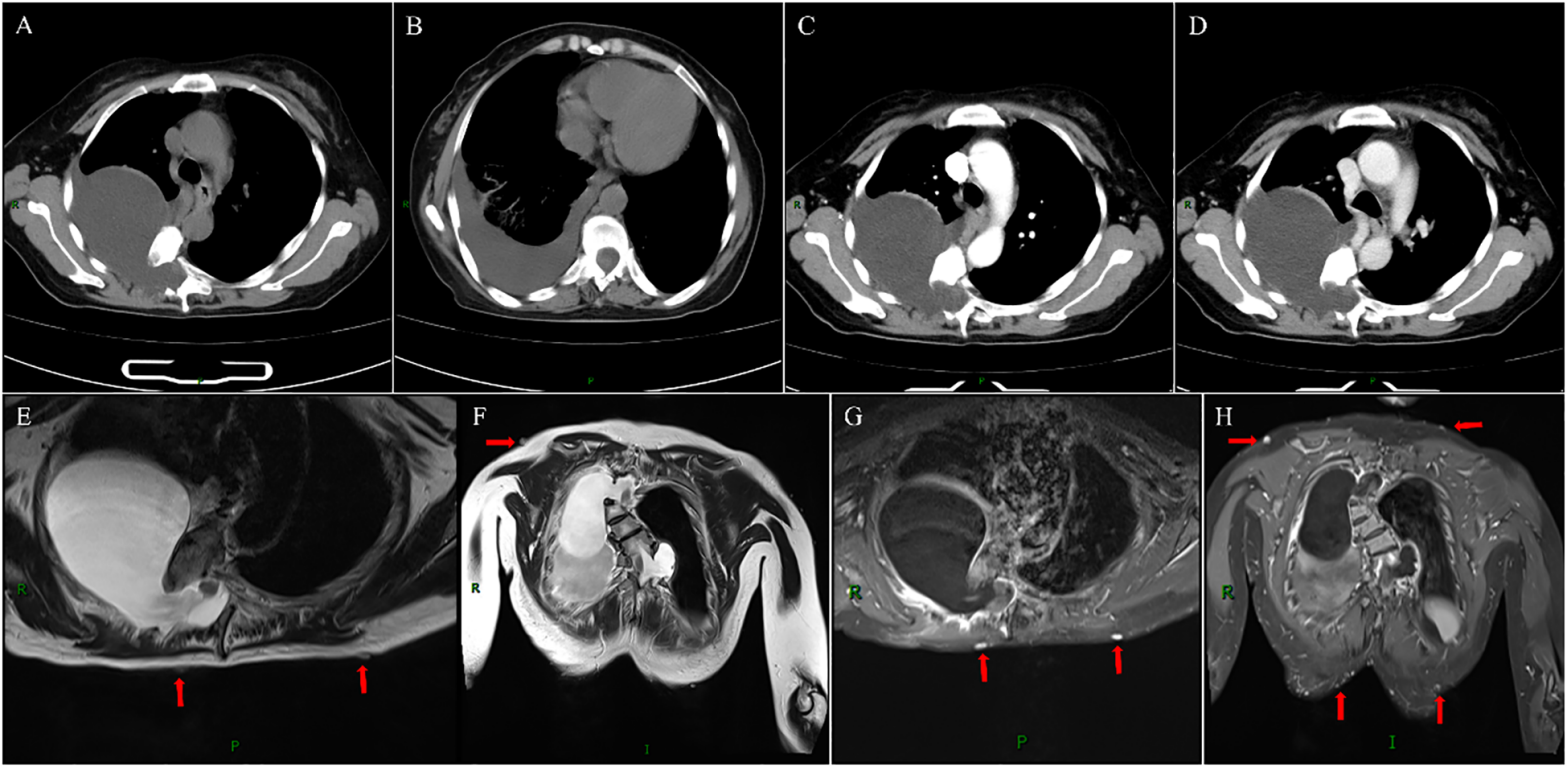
Imaging findings from April 2023. Non-contrast and contrast-enhanced chest CT demonstrates significant enlargement of the right T5/T6 foraminal meningocele compared to 2015, with no internal enhancement. New right-sided pleural effusion is present (A-D). Post-thoracentesis thoracic spine MRI (non-contrast + contrast) reveals a homogeneous T2-hyperintense meningocele at the right T5/T6 foramen. Contrast-enhanced sequences show no internal enhancement of the lesion but prominent posterior wall enhancement, potentially related to the puncture procedure. Additionally, multiple enhancing cutaneous and subcutaneous nodules are noted on MRI (red arrows) (E-H).
